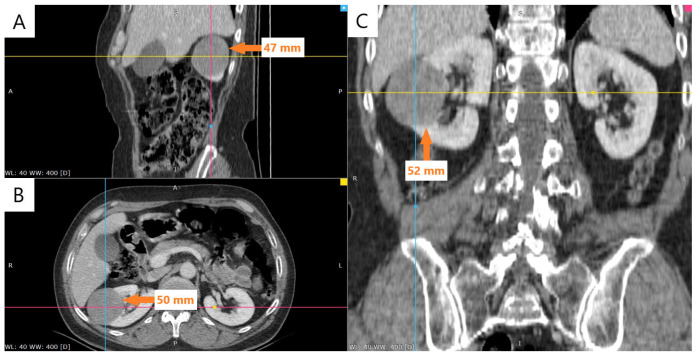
Contrast-enhanced CT in the venous phase (60 s), multiplanar reconstruction (MPR). (A) Axial image showing a well-defined nodular lesion in the mid-portion of the right kidney, measuring 47 mm (arrow). (B) Axial image with a maximum diameter of 50 mm (arrow). (C) Coronal image showing craniocaudal extension of 52 mm (arrow).
